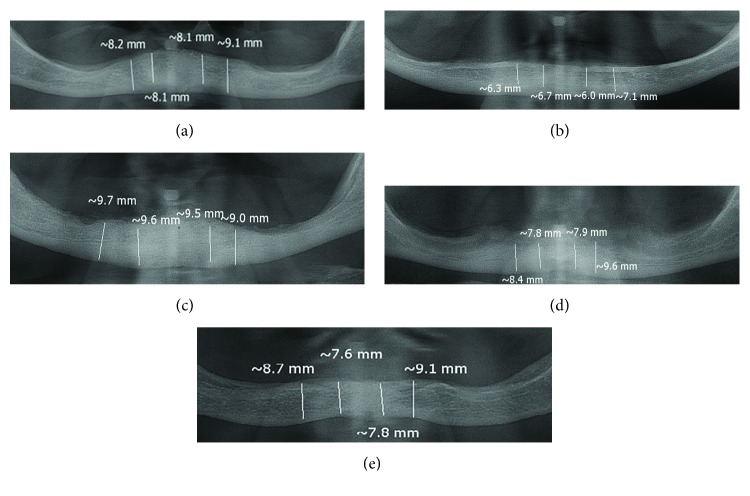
(a–e) Measurement of interforaminal mandibular height on panoramic radiographs of atrophied mandibles using the software SCANORA.
